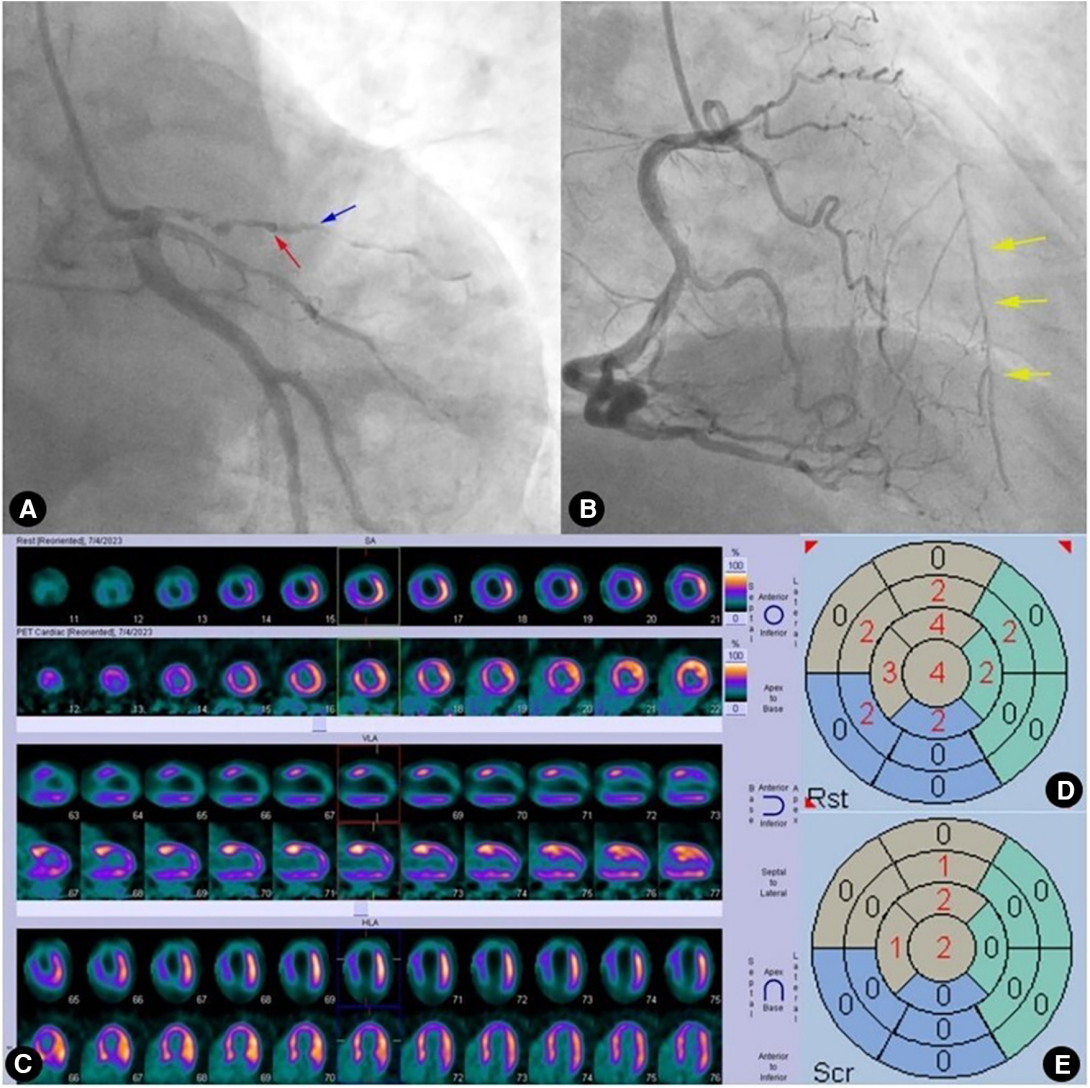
Case 2: male, 59 years old. (A) Coronary angiography showed that the left anterior descending artery (LAD) was completely occluded (red arrow) after emitting a diagonal branch (blue arrow). (B) Coronary angiography showed WD (Rentrop grade 2) from the right coronary artery (RCA) to the LAD (yellow arrow). (C) Rows 1, 3, and 5 presented ^99m^Tc-MIBI SPECT MPI, demonstrating segmental radiotracer deficits/defects in parts of the anterior wall, septal wall, and apex (five myocardial segments supplied by the LAD). Rows 2, 4, and 6 presented ^18^F-FDG PET MMI. The segments showing radiotracer deficits/defects in the perfusion imaging exhibited noticeable radiotracer uptake in the metabolic imaging, indicating a perfusion/metabolism “mismatch”. (D) Scoring for myocardial perfusion imaging. (E) Scoring for myocardial metabolism imaging. After calculations, the HM index is (15-6)/(5 × 4) × 100% = 45%.
